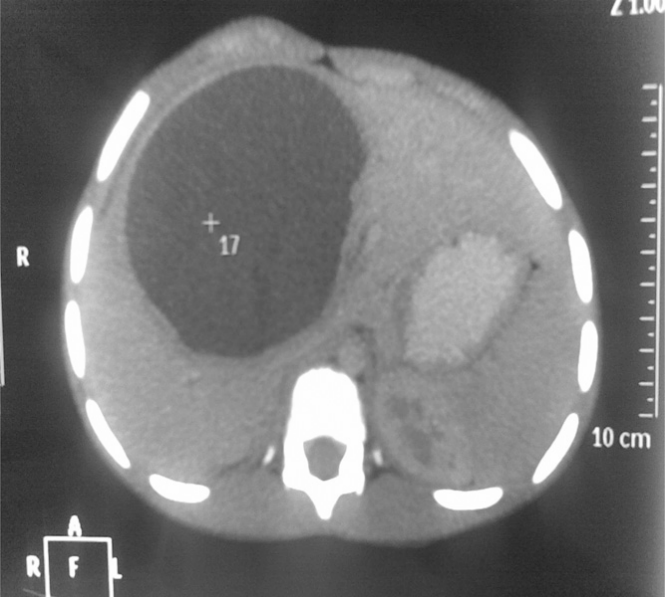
Image from a computed tomography study of the abdomen of the patient.
